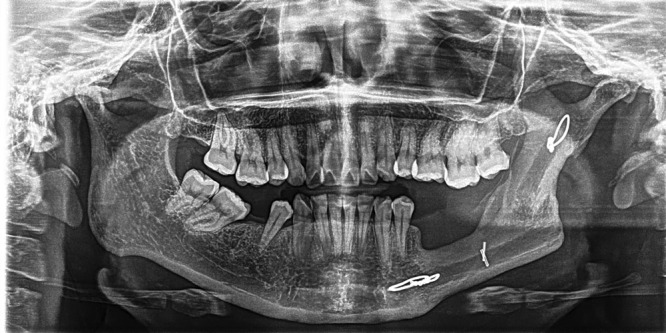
Orthopantomography at 8 years showing spontaneous bone remodeling.
